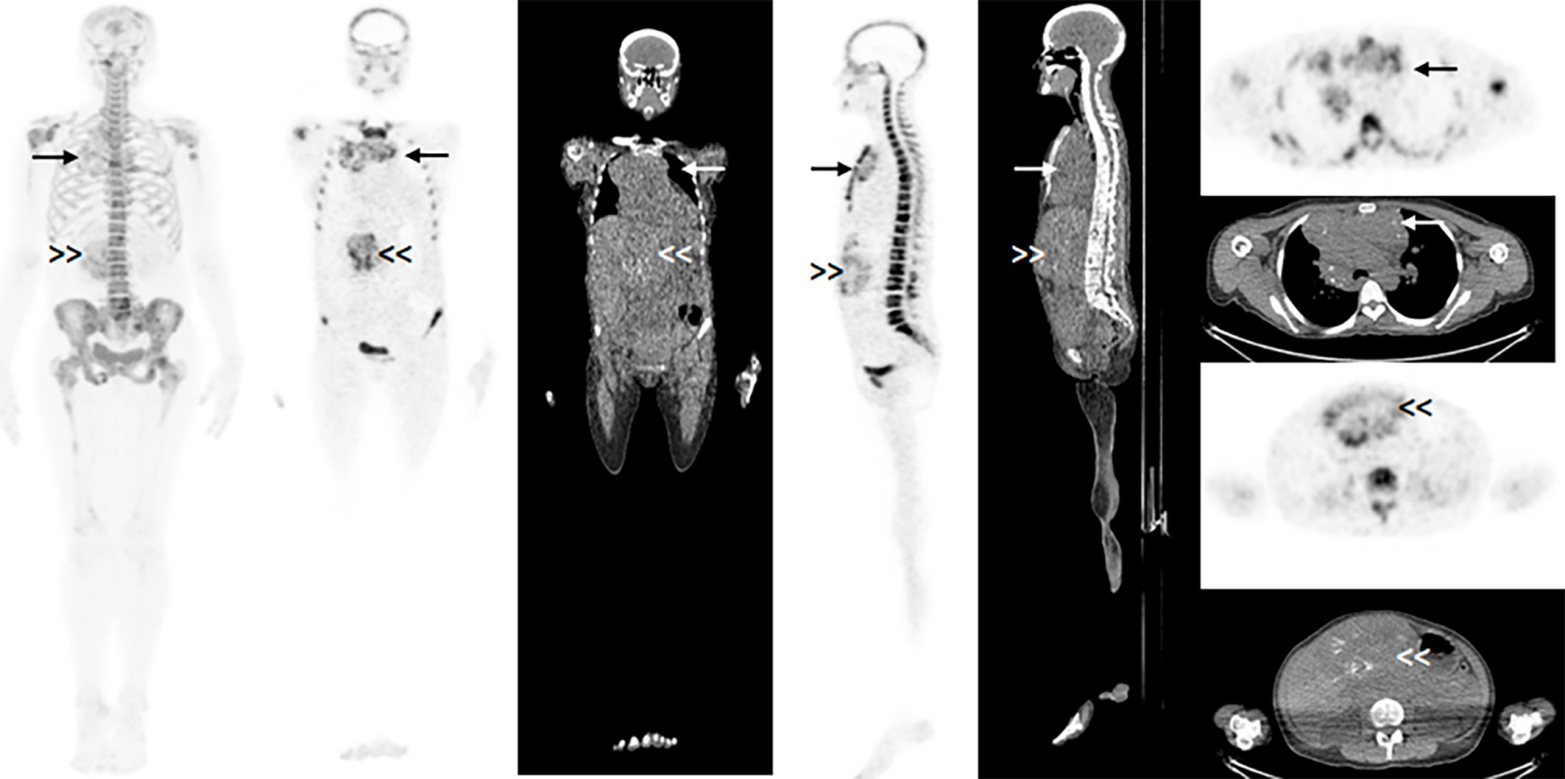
^18^F-NaF PET/CT study showing multiple bone metastatic lesions and extraosseous disease in the liver (double arrowhead) and mediastinum (arrow). Maximal intensity projection (MIP) coronal image (on the left side of the figure) and coronal, sagittal, and axial images of the metabolic and anatomical parts of the study at the level of the extraosseous lesions.
